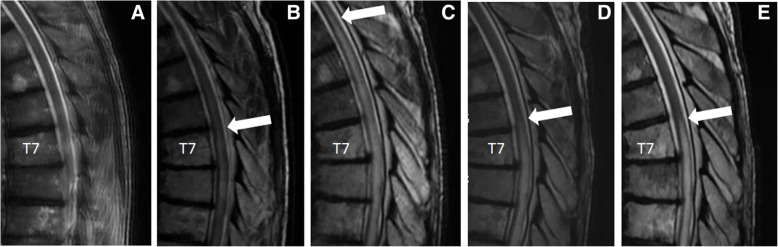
T2-weighted sagittal MRI images of thoracic spine. The 7th thoracic vertebrae is marked for reference. Arrow indicates the superior border of the transverse myelitis. a before initiation of therapy, no intrinsic spinal cord lesion. b 6 months after radiation, with cord changes around T7. c 11 months after radiation, with progressive symptoms and ascending transverse myelitis despite therapy. d Three weeks after initiation of infliximab, with significant improvement. e. Two months after infliximab, still with some cord abnormality around T7
